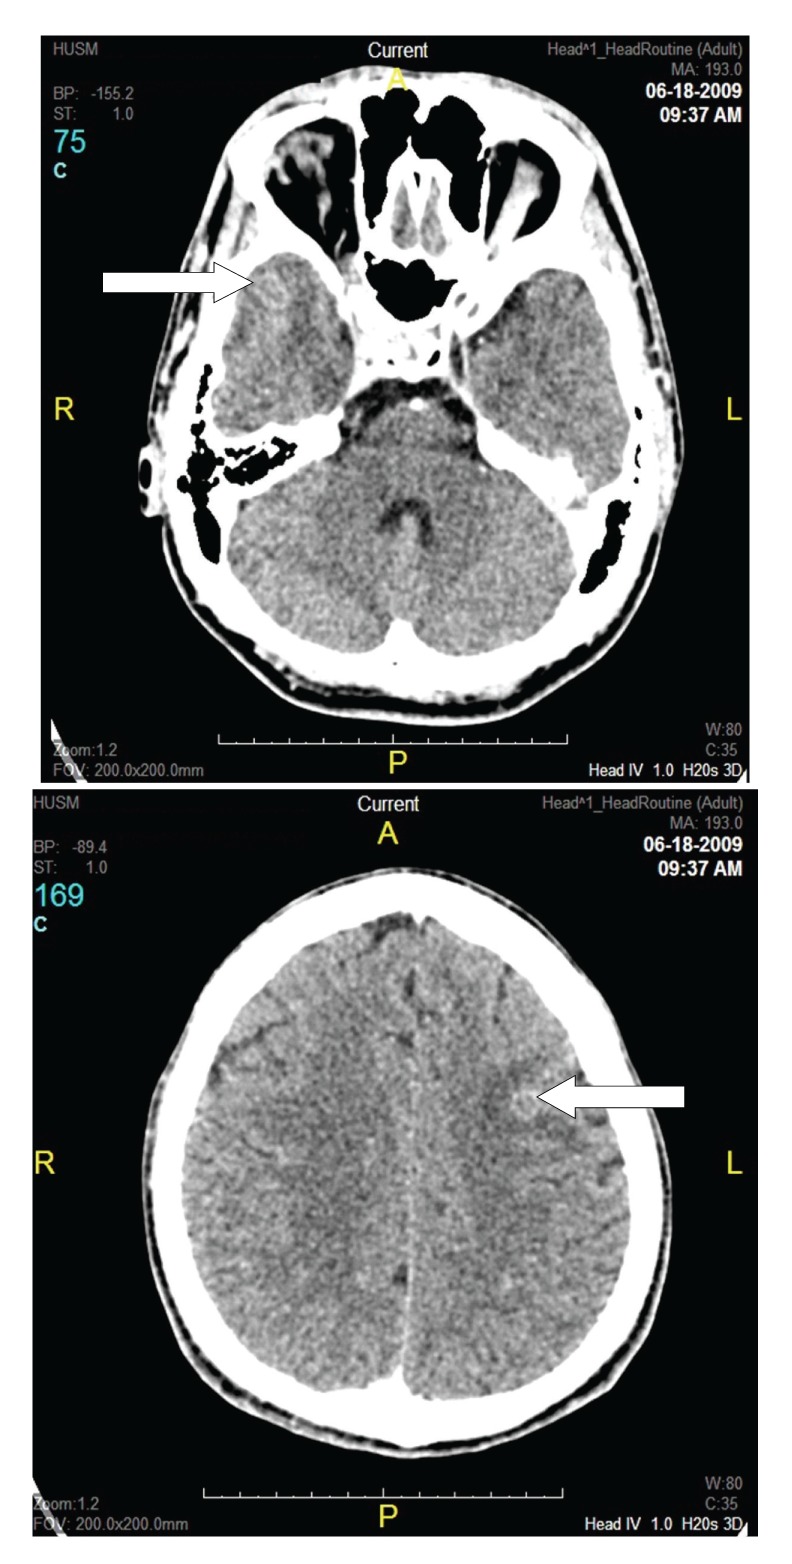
CT scan of brain and orbit showed ill-defined peripherally enhancing iso to hypodense lesions with perilesional edema at right frontal and left temporal lobe consistent with cerebral abscesses.
